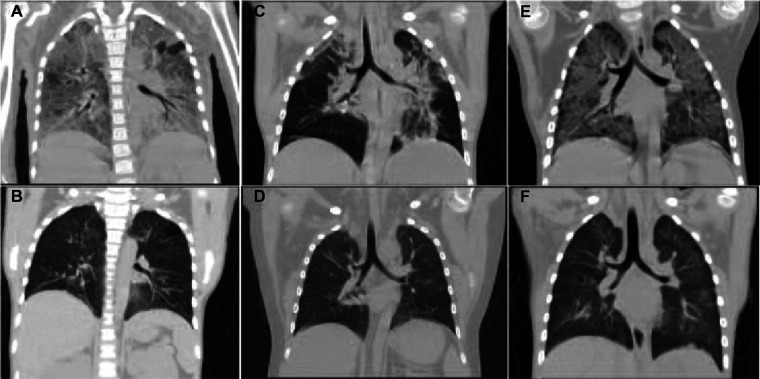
The CT images of lungs in three PNS cases with PCP. It showed that extensive exudation in both lungs was improved significantly after antifungal treatment. (A,B) The CT images of lungs before and after anti-fungal treatment in Case 1. (C,D) The CT images of the lungs before and after treatment in Case 2. (E,F) The CT images of lungs before and after treatment in Case 3.
